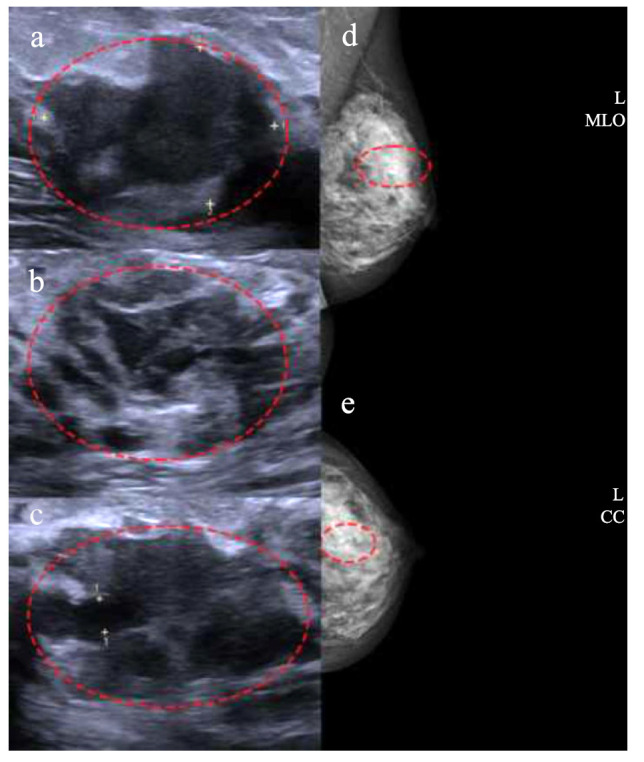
Ultrasound and X-ray images of a 40-year-old woman with primary mucinous cystadenocarcinoma (MCA) combined with invasive ductal carcinoma (IDC) in the left breast. (a) Ultrasonography revealed a mixed echo area in the upper outer quadrant of the left breast, measuring approximately 60 × 17 × 40mm. The lesion displayed unclear borders and an irregular shape, with an irregular liquid dark area in the center and a lack of internal sound conduction. (b) Multiple ductal dilations were observed surrounding the lesion. (c) The dilated part of the duct measured about 4 mm and appeared connected with the mixed echo area. (d, e) Mammography Mediolateral Oblique (MLO) images and craniocaudal (CC) images depicted amorphous suspicious calcifications in the middle of the upper outer quadrant of the left breast, distributed segmentally. (The area within the red circle indicates the lesion area).
